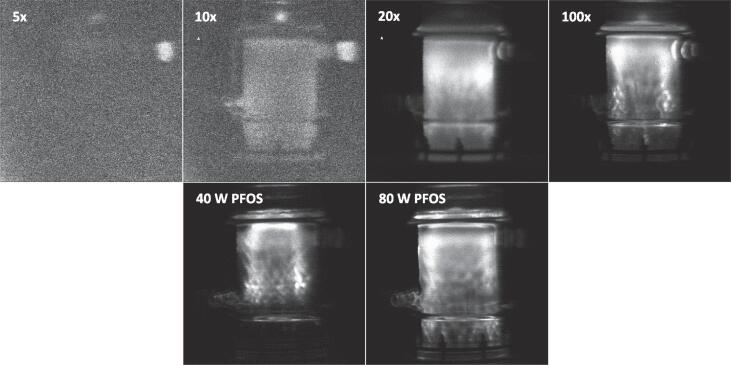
SL images of diluted 3 M Lightwater (above) and 10.0 mg/L PFOS (below). The images were captured using an EM gain of 50 s, exposure time of 60 s, (5×, 10×, and 20 × ) or 15 s (100 × and both PFOS images). The ultrasound was set to 80 W and 410 kHz for all bar the “40 W PFOS” image.
